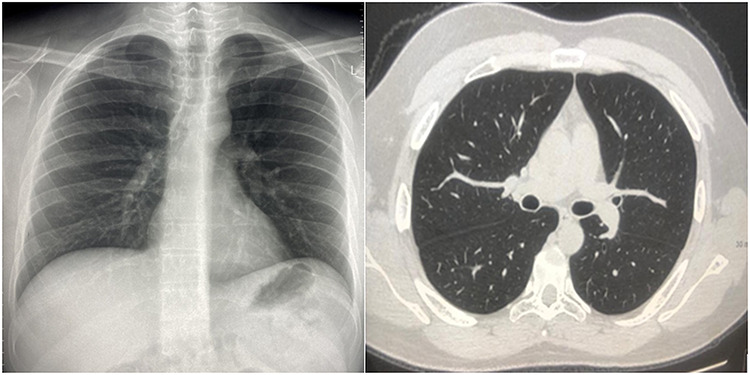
Antero-posterior view of chest radiograph (left) showing normal lung fields and CT of the chest (right) confirming absence of parenchyma lung involvement.
